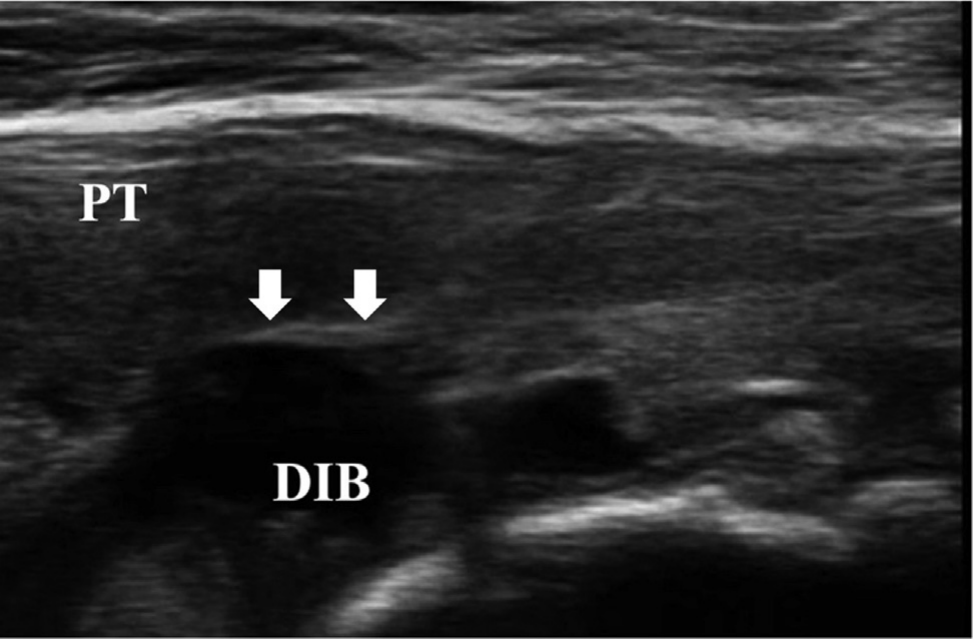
Ultrasound image of the patient’s left knee after ossicle removal. The area is inspected again by ultrasound to confirm the absence of remnant ossicles, The white arrows indicate where the ossicle is located. (DIB, deep infrapatellar bursa; PT, patellar tendon.)
